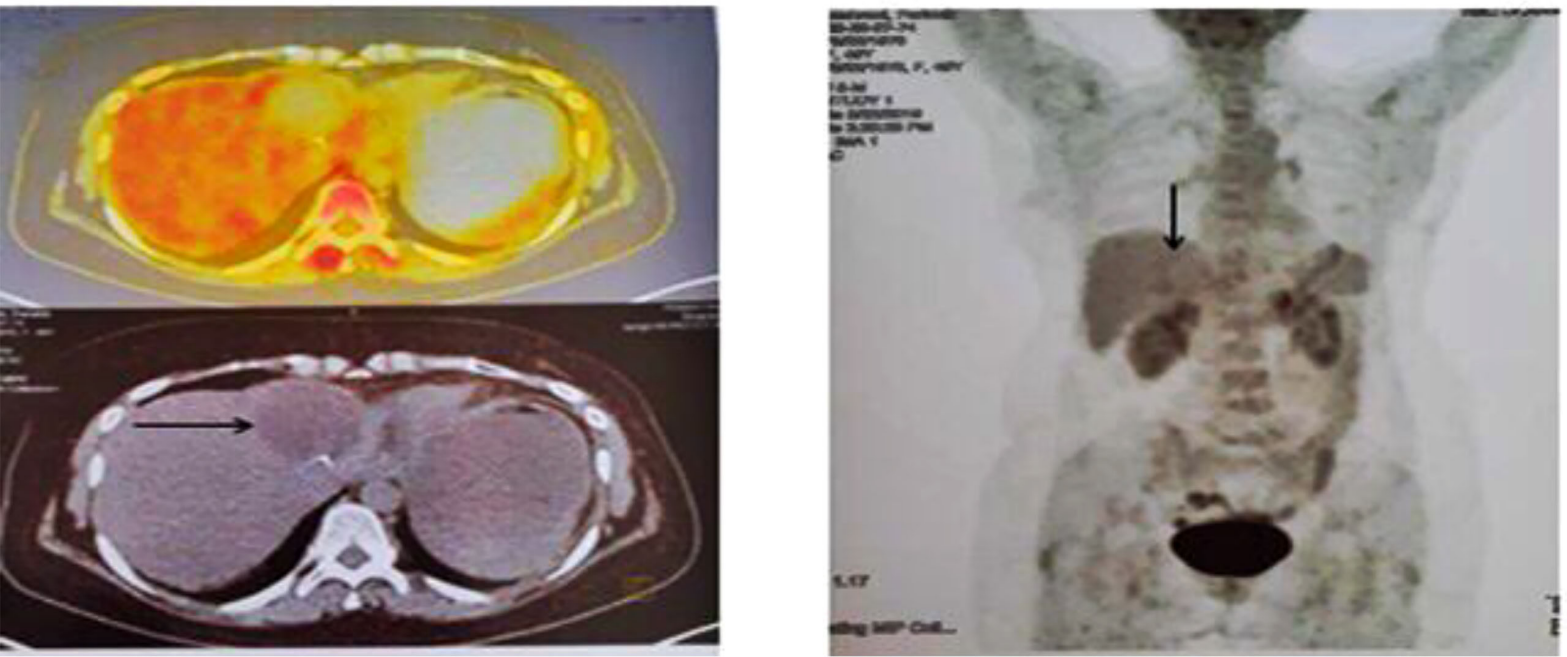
Second PET/CT on 23 May 2019. When compared with prior FDG PET/CT scan, the patient status post left liver lobe segment 4 resection, with non-FDG-avid post-surgical change in the surgical bed, which was compatible with an excellent complete metabolic response to therapy.
